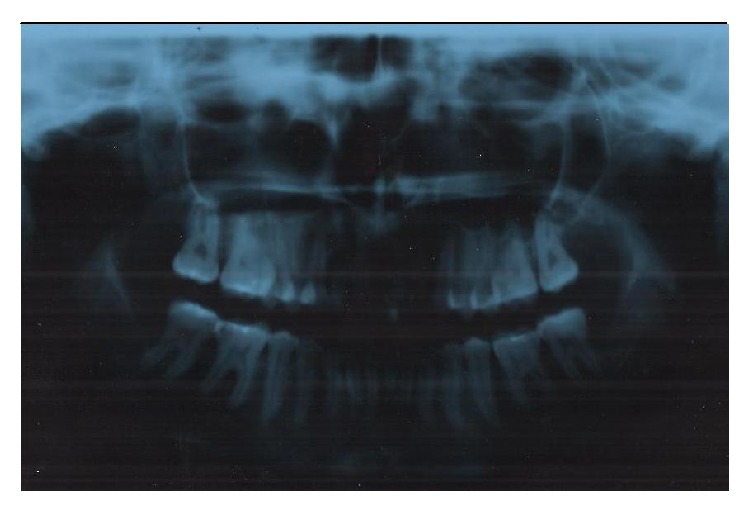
X-ray for postoperative evaluation.
